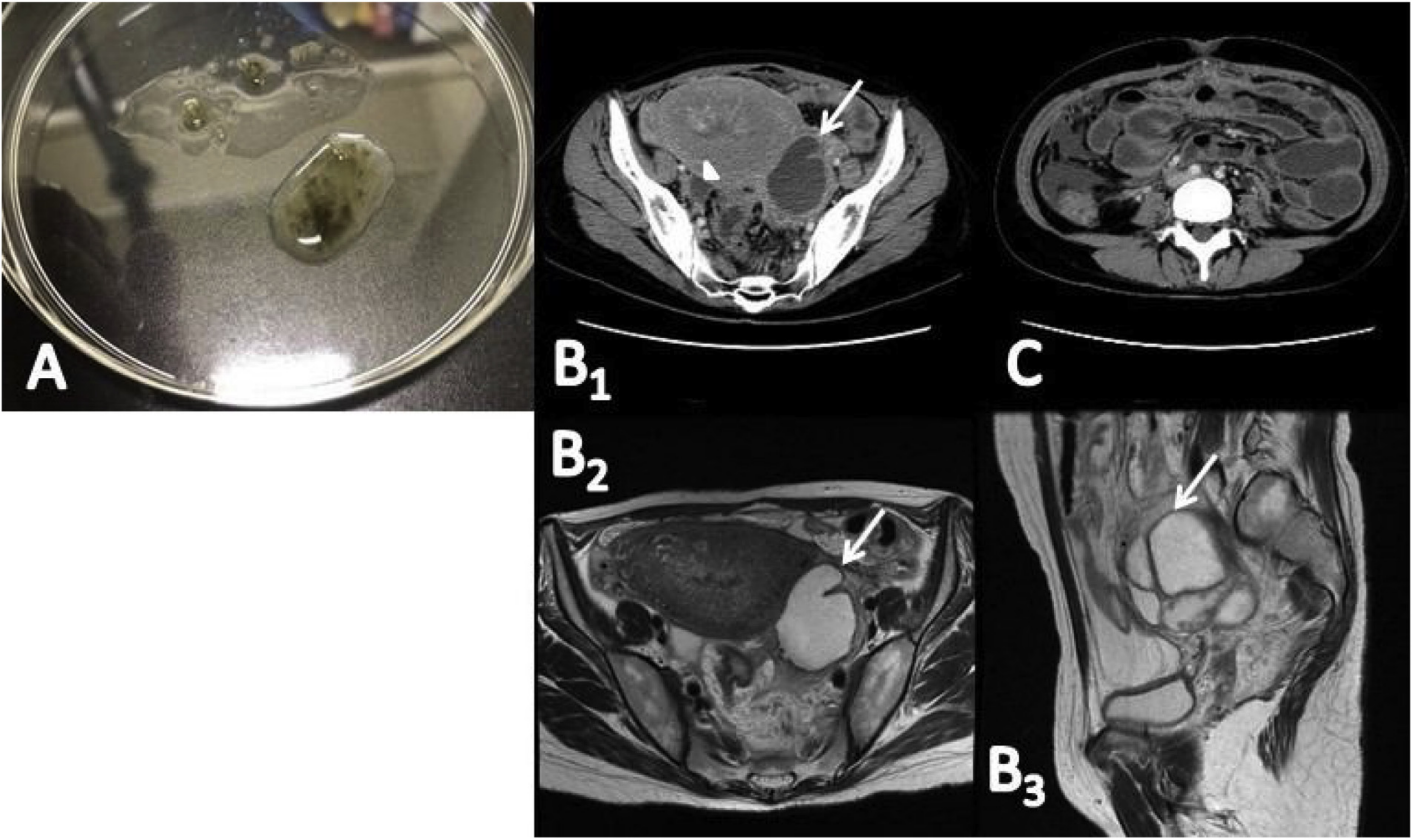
Stool and CT findings. (A): A sample of greenish diarrhea. (B_1-3_): CT and magnetic resonance images showing a left adnexal mass (arrows) and fluid collection (arrow) in the pelvis. (C): CT image also showing wall thickness and fluid collection in the small intestine. CT, computed tomography. (For interpretation of the references to colour in this figure legend, the reader is referred to the web version of this article).
